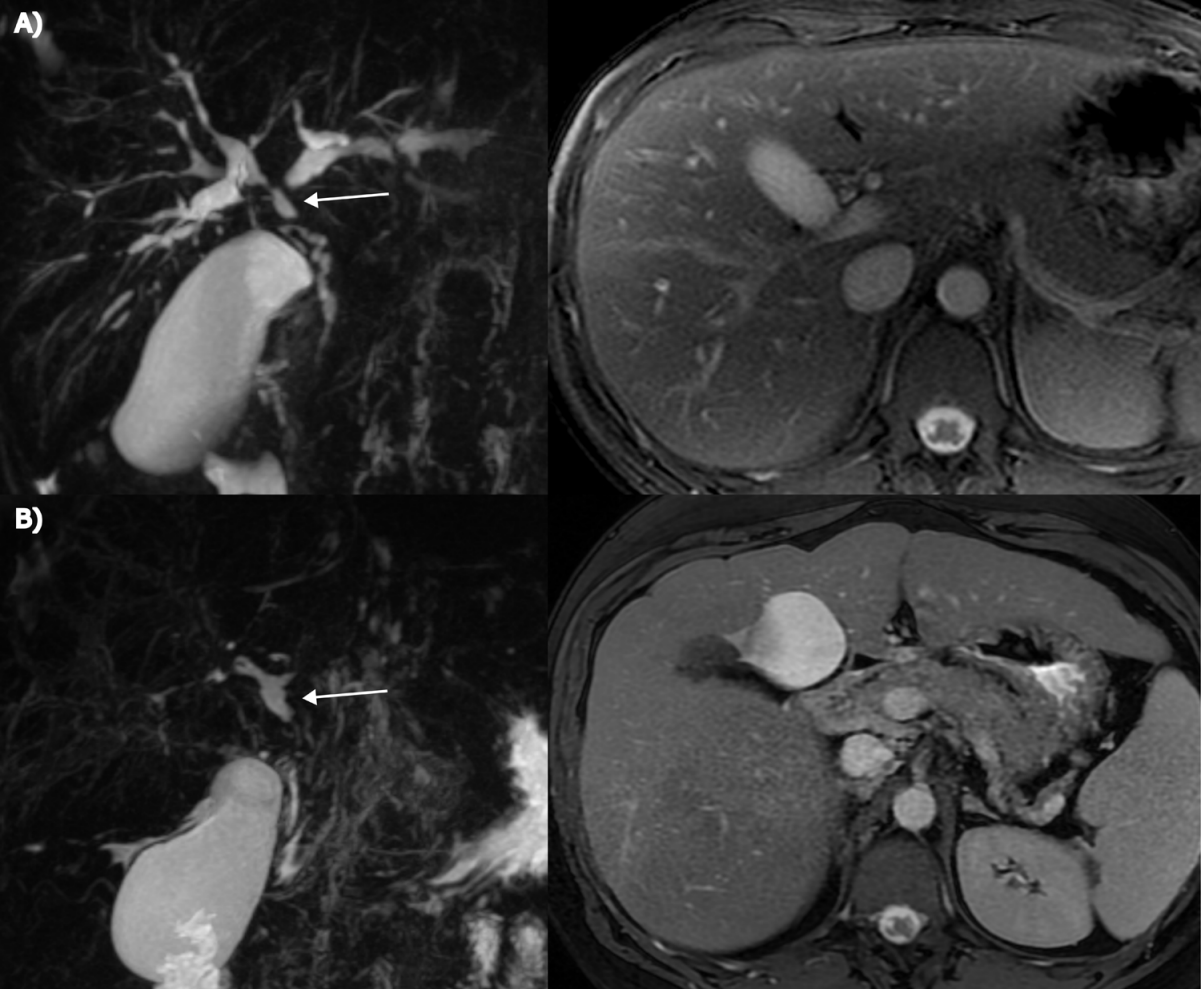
An example of a counter-intuitive behavior of ANALI subscores. (a) Patient presenting with marked IHD dilatation and normal liver morphology on initial MRI (ANALI_NoGd_: 2 + 0 + 0 = 2). (b) After an 8-year follow-up period, the IHD dilatation had decreased, while extensive biliary stricturing with a pruned-tree appearance had developed, accompanied by progressive hepatic dysmorphia and the formation of collateral vessels (not shown). Despite noticeable disease progression, the ANALI_NoGd_ score increased only to 3 due to diminished IHD dilatation (ANALI_NoGd_: 0 + 2 + 1 = 3). For anatomical orientation, the common hepatic duct is indicated by an arrow in the figures. IHD, intrahepatic bile duct.
